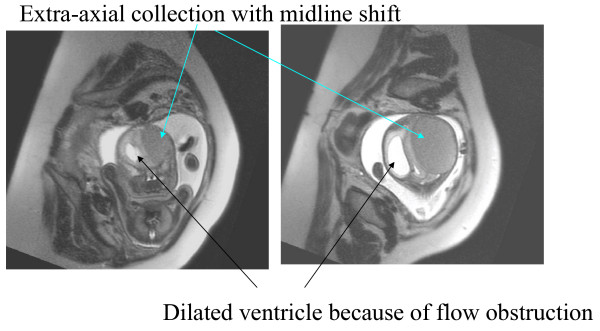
Fetal MRI, T2 weighted coronal section with a mass effect on the right side with mid-line shift to the left side because of sub-dural bleeding.
